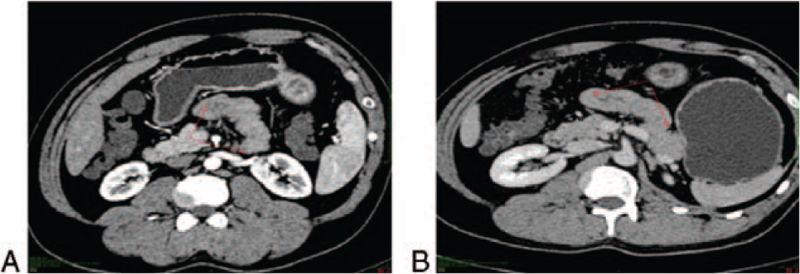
Enhanced CT images. The images show diffuse thickening of the jejunum wall (arrows), which was suspected to be inflammation, although the presence of a tumor could not be ruled out completely. CT = computerized tomography.
